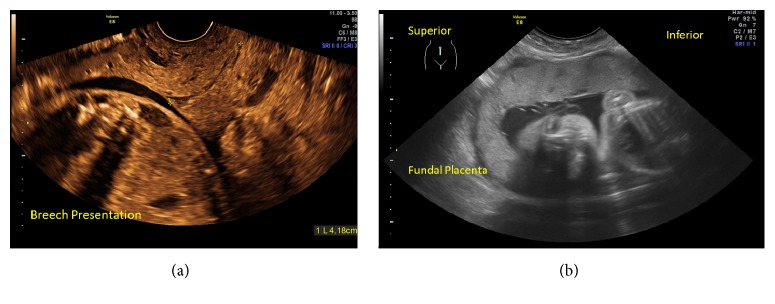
(a) Endovaginal ultrasound at 26 weeks showing a normal cervical length of 4.16 cm. (b) Transabdominal ultrasound showed a fundal placenta with breech presentation.
